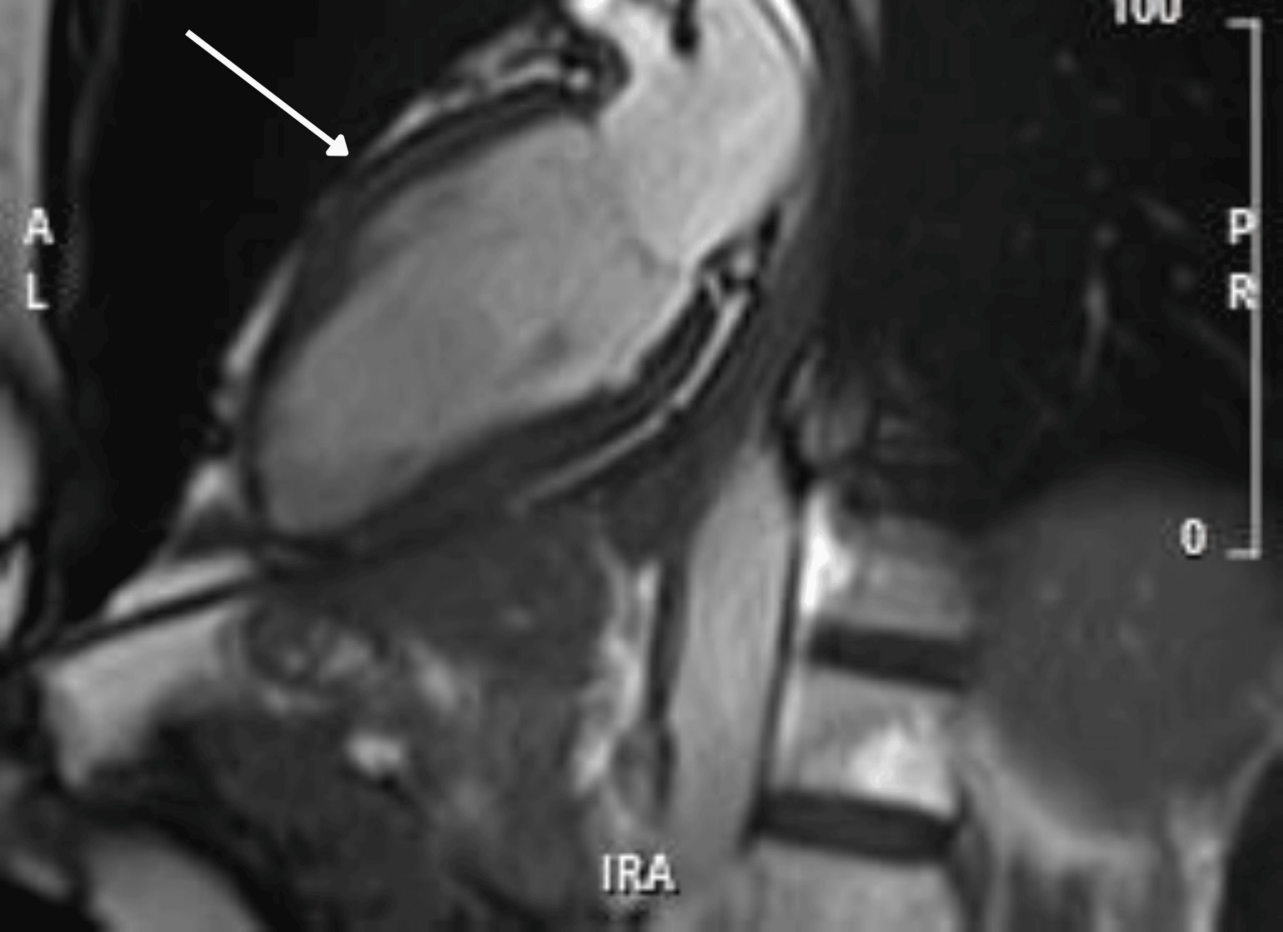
Cardiac MRI showing left ventricular diastole MRI: magnetic resonance imaging
